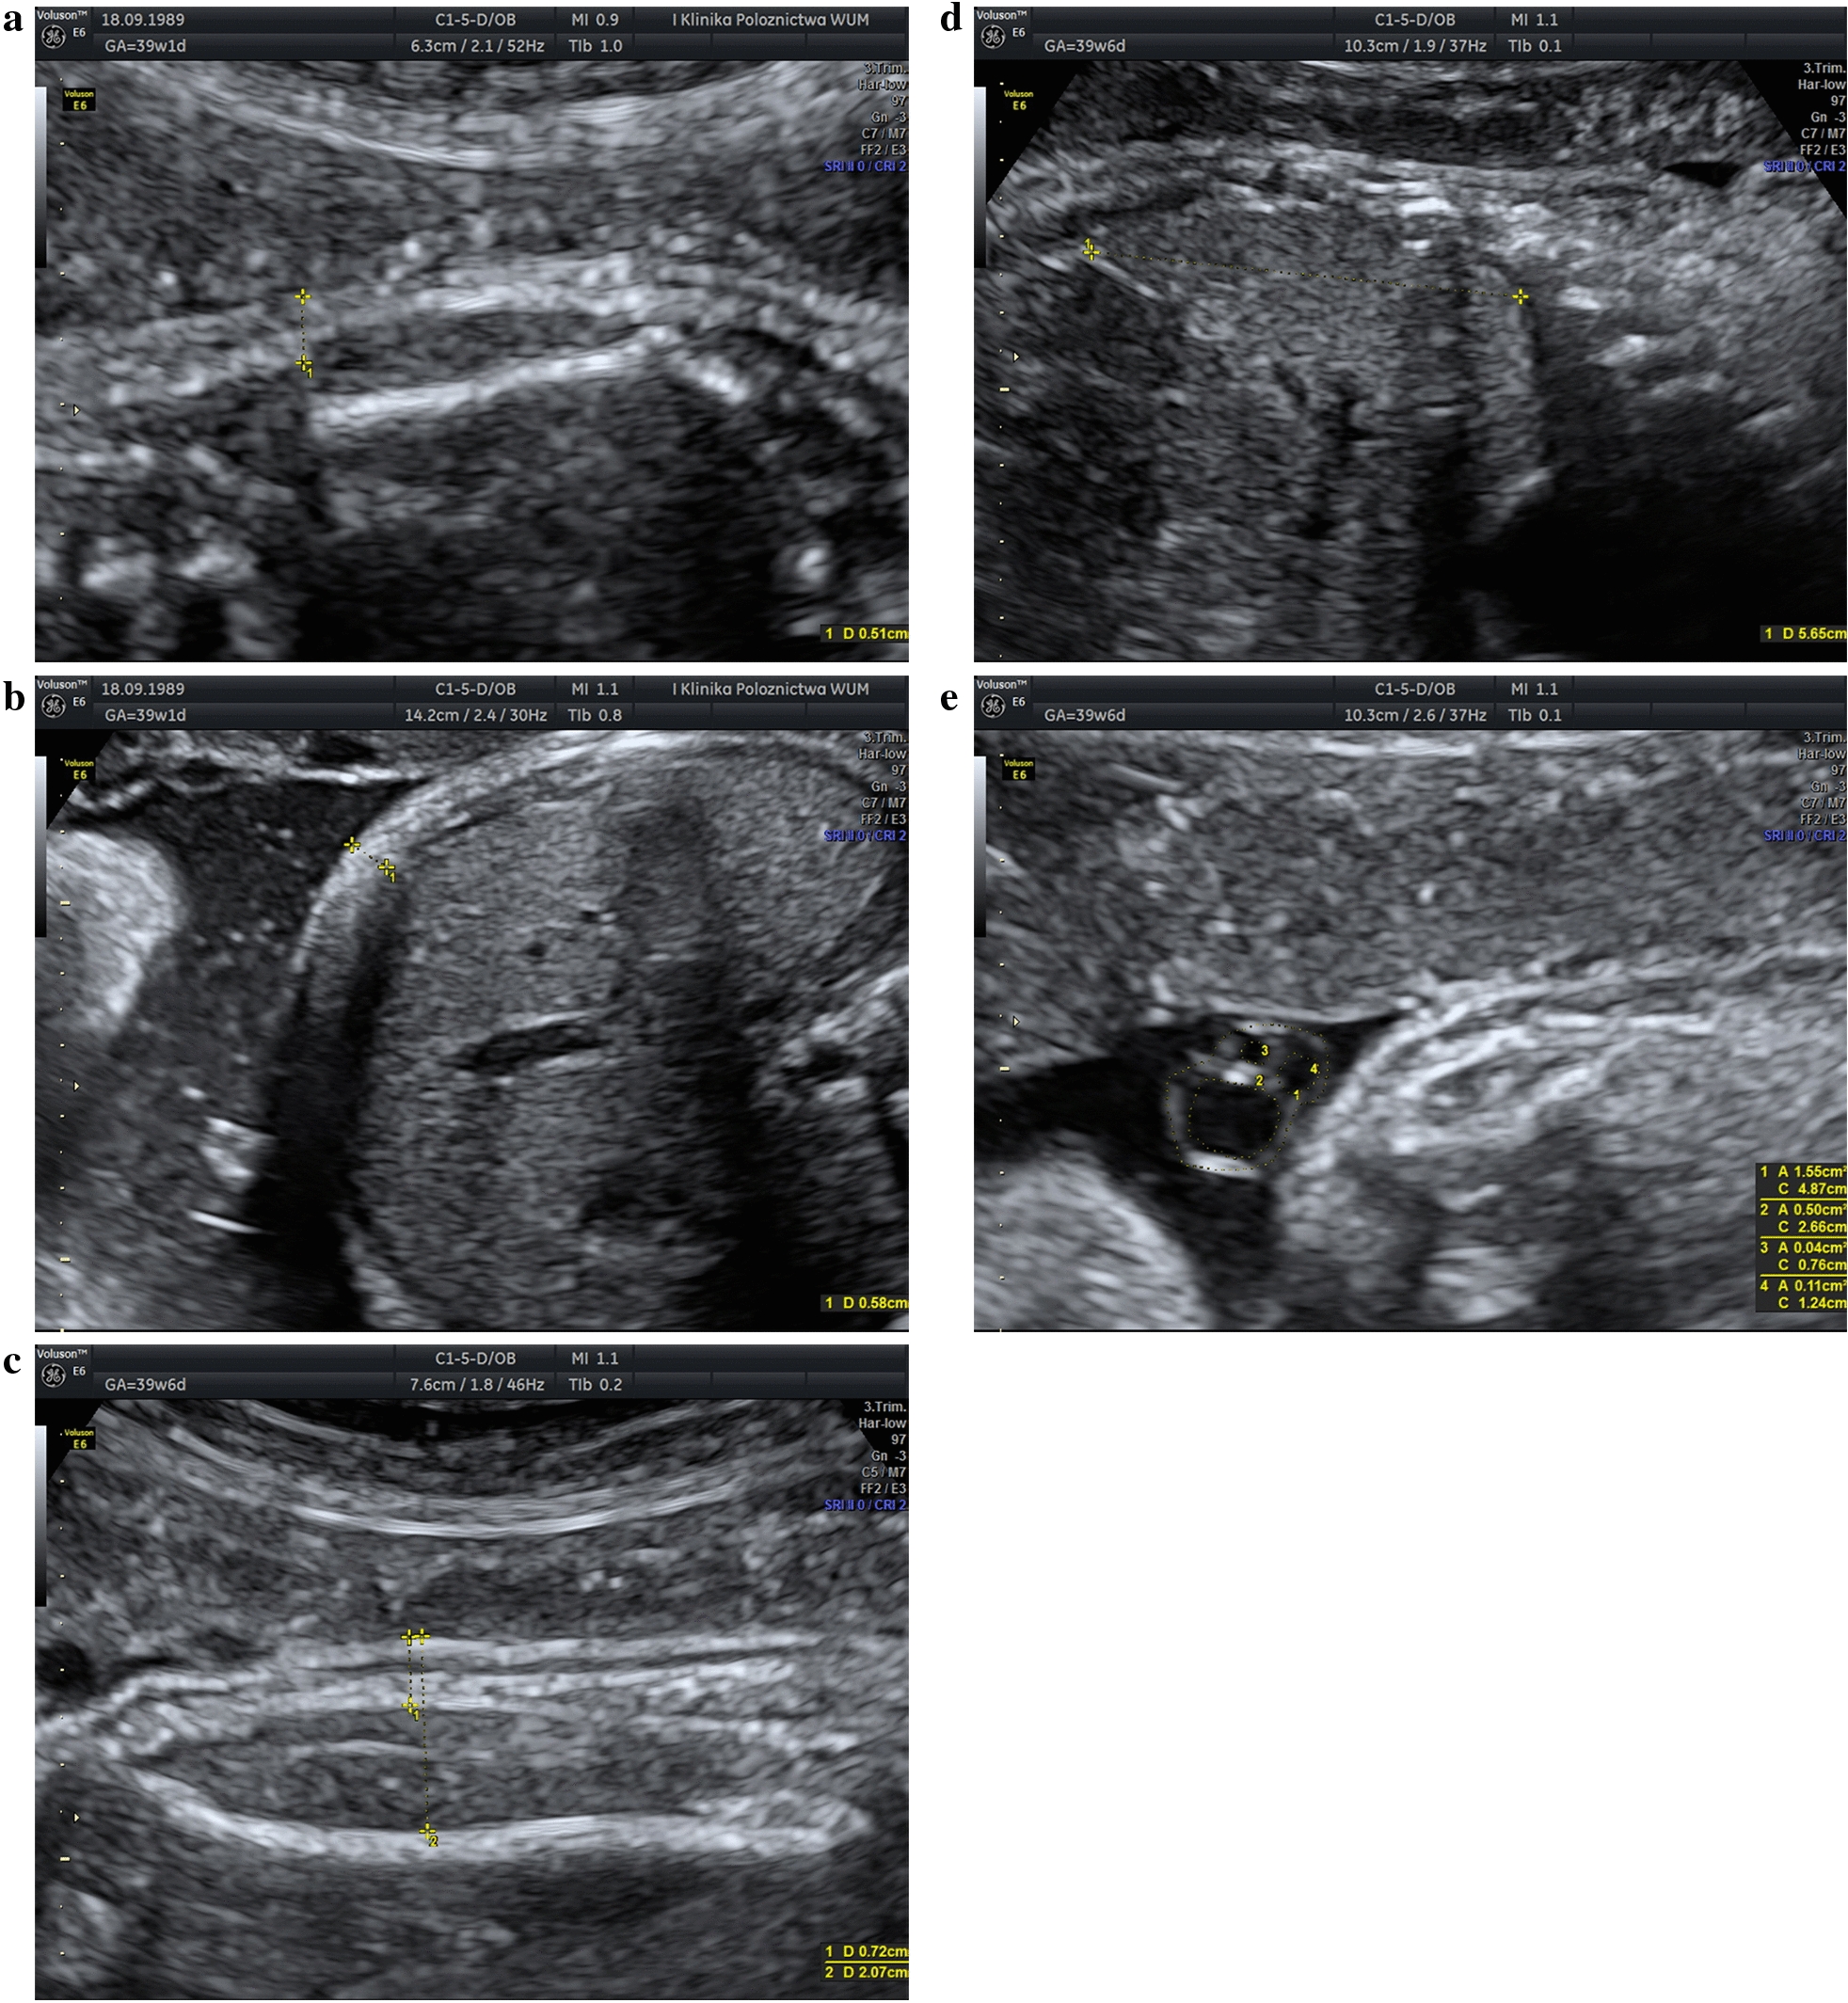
Ultrasound images presenting fetal sub-scapular fat mass (a), abdominal fat mass (b), mid-thigh fat mass/mid-thigh lean mass (c), liver length (d), umbilical cord/umbilical vein/umbilical artery circumference and area (e) measurement technique
